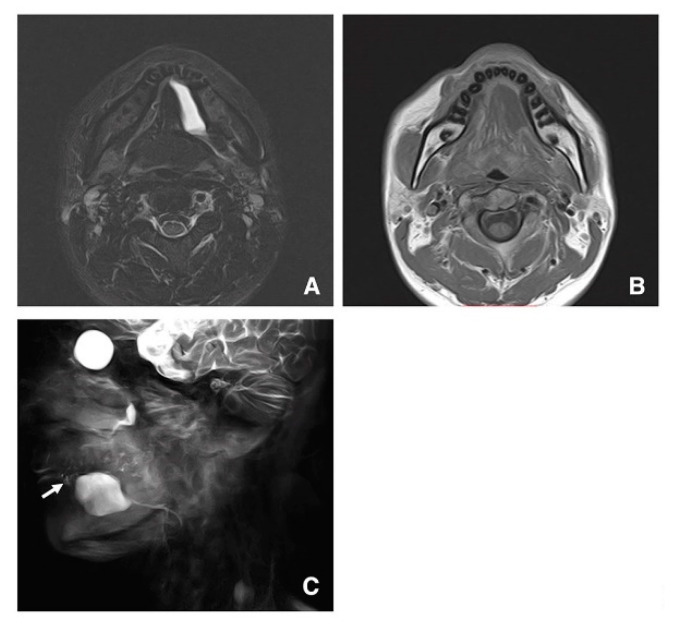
STIR (A), T1-weighted image (B), and MR sialography (C) of a 30-year-old woman with a ranula on the left. The mass lesion is seen in continuity with the sublingual glands in STIR (A) and T1-weighted images (B) and is diagnosed as a ranula. The mass is derived from one of many sublingual gland ducts (arrow) (C).
